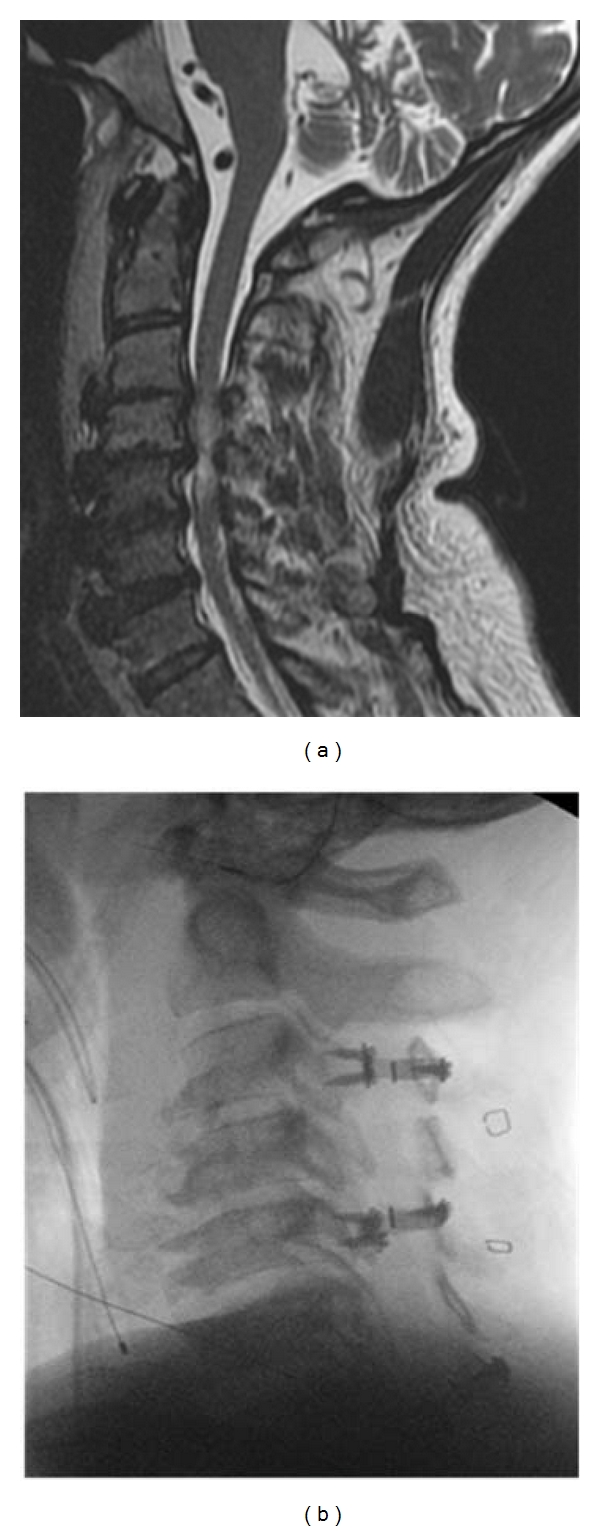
(a) Sagittal MRI of a patient with multilevel stenosis and preserved lordosis. (b) Lateral radiograph of same patient after canal-expanding laminoplasty.
